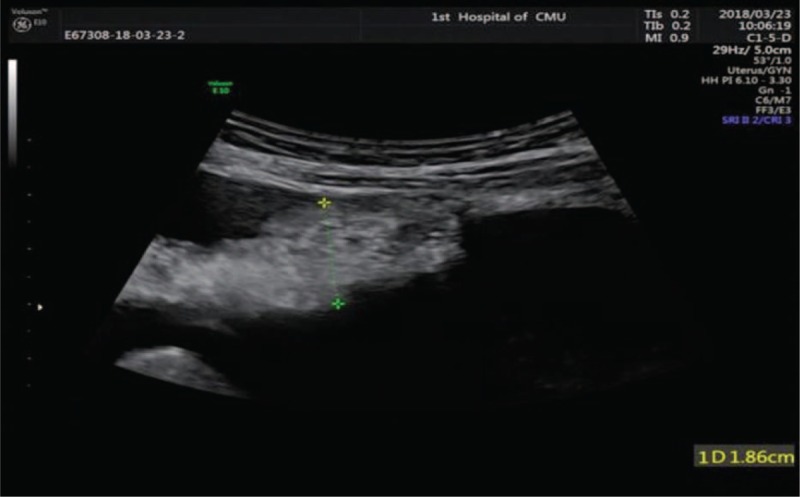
Peritoneal ultrasound shows a thickened uneven echo peritoneum in the left lower abdomen.
